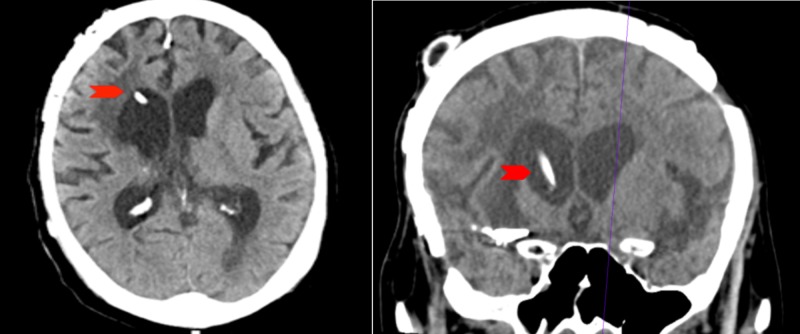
CT of the head showing VP shunt entering anterior horn of right lateral ventricle (red arrow) CT, computed tomography; VP, ventriculoperitoneal
